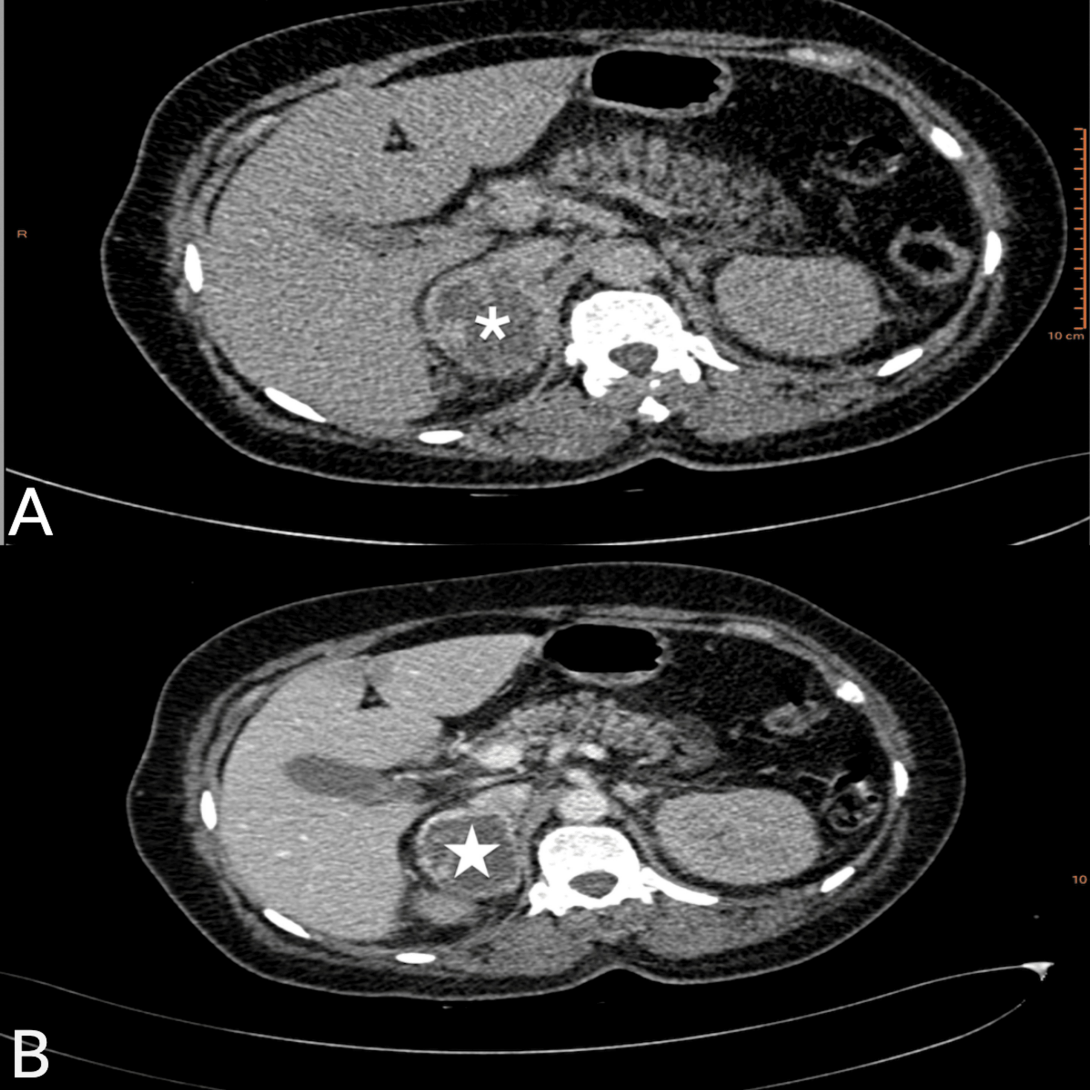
CT scan before (A) and after contrast injection (B) revealed a right adrenal mass (white star) of 4 cm, solid-cystic, with the tissue component enhancing after contrast injection.
